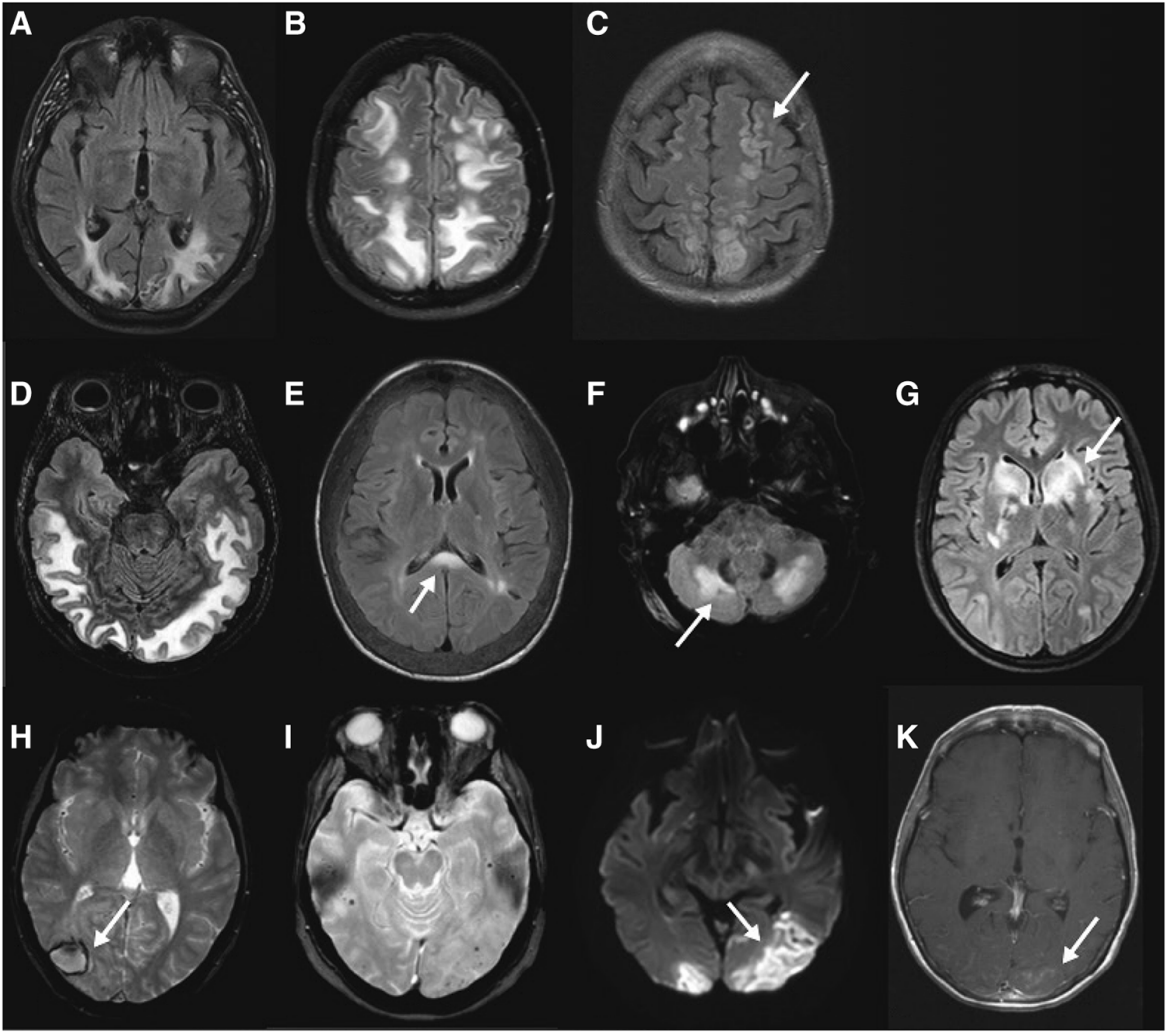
PRES patterns on MRI brain imaging. a FLAIR dominant parieto-occipital pattern, b FLAIR holohemispheric watershed pattern, c FLAIR sulcus frontalis superior (*arrow*) and holohemispheric watershed pattern, d temporal lobe pattern, e splenium corporis callosi pattern, f cerebellar pattern, g basal ganglia pattern. h GE (gradient echo) macrohaemorrhage, i GE multiple microhaemorrhages, j diffusion-weighted restricted diffusion, k contrast enhancement
